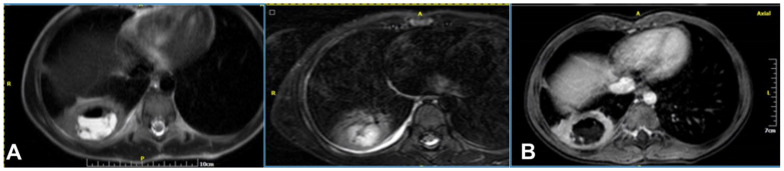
A 6-year-old boy, referred to MRI for exclusion of a lung abscess. (A) T2-weighted sequence shows a right lower lobe consolidation with a single air–fluid cavity (area with high and very low signal intensity), without a clearly depicted wall (area of isointensity surrounding the cavity) and an adjacent small pleural effusion (crescent-shaped line of high signal intensity). (B) Post-contrast T1-weighted sequence shows contrast enhancement by the adjacent consolidated lung parenchyma, consistent with the presence of cavitary necrosis.
